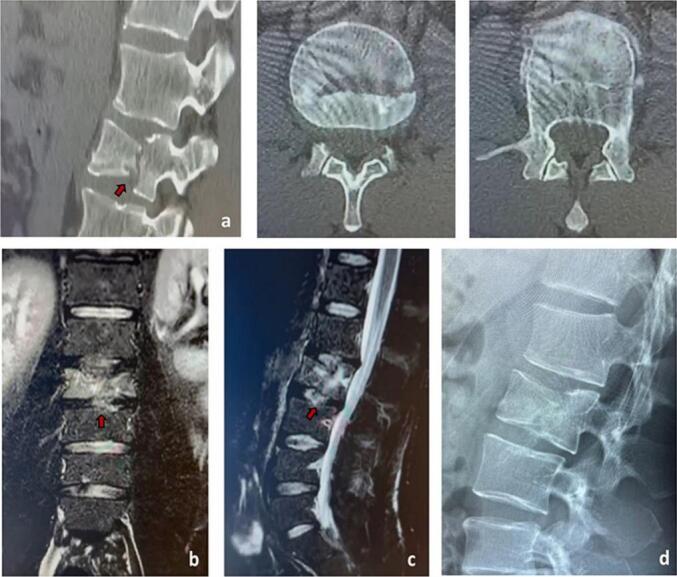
a) Computed Tomography (CT) scan at 40 days after the trauma (sagittal and transverse views), further showing L2 vertebral compression fracture with no improvement and no bone healing (red arrow). b-c) Magnetic Resonance Imaging (MRI) at 40 days after the trauma (coronal and sagittal views) showing comminuted L2 vertebral fracture and bone edema around the fracture. d) Radiography at 40 days after the trauma (sagittal view), showing L2 vertebral fracture. (For interpretation of the references to colour in this figure legend, the reader is referred to the web version of this article.)
